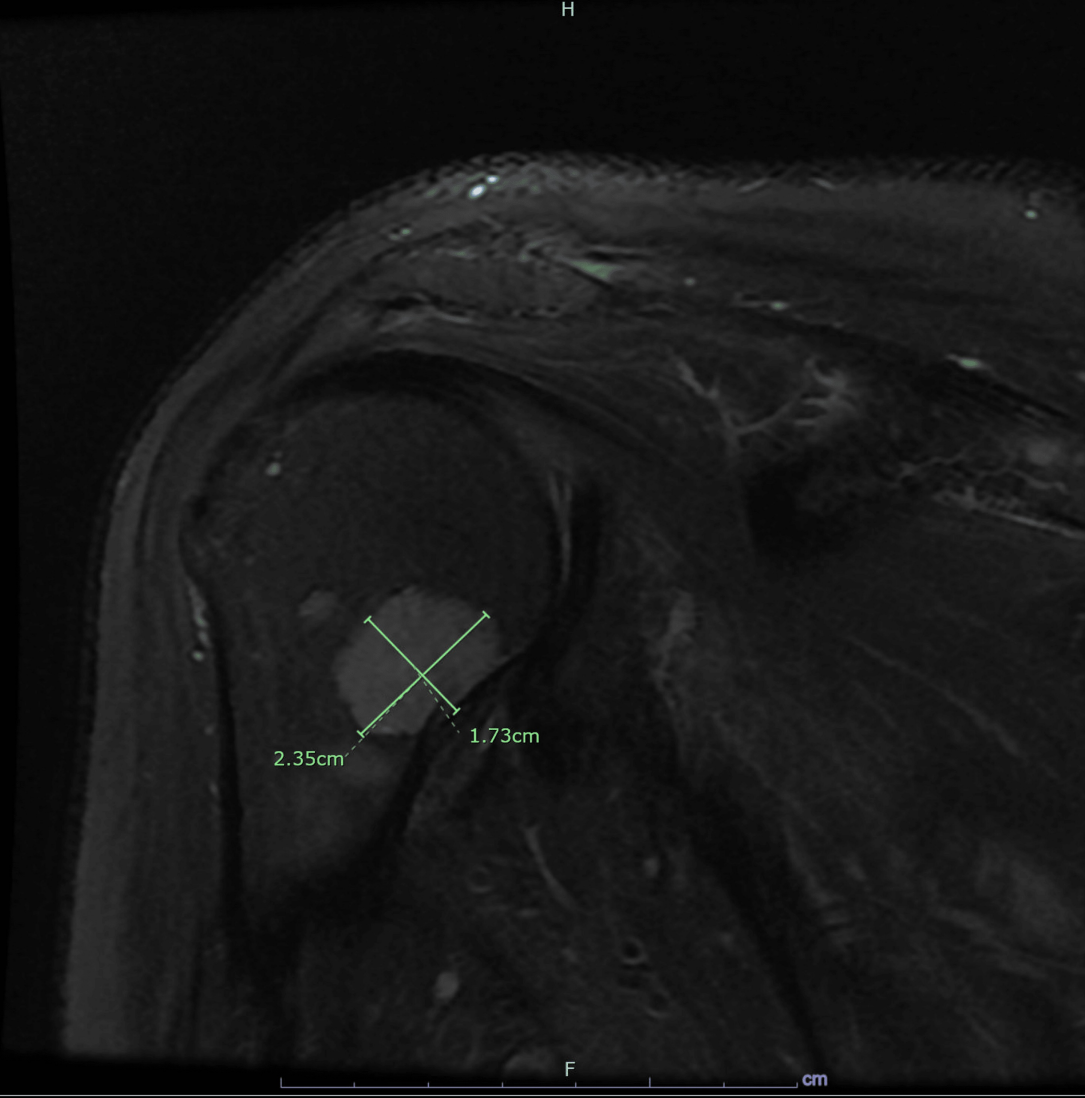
MRI without contrast of the left shoulder, left proximal humerus Additional smaller masses are seen within the proximal humerus with the largest measuring 1.7 x 2.4 cm MRI: magnetic resonance imaging
